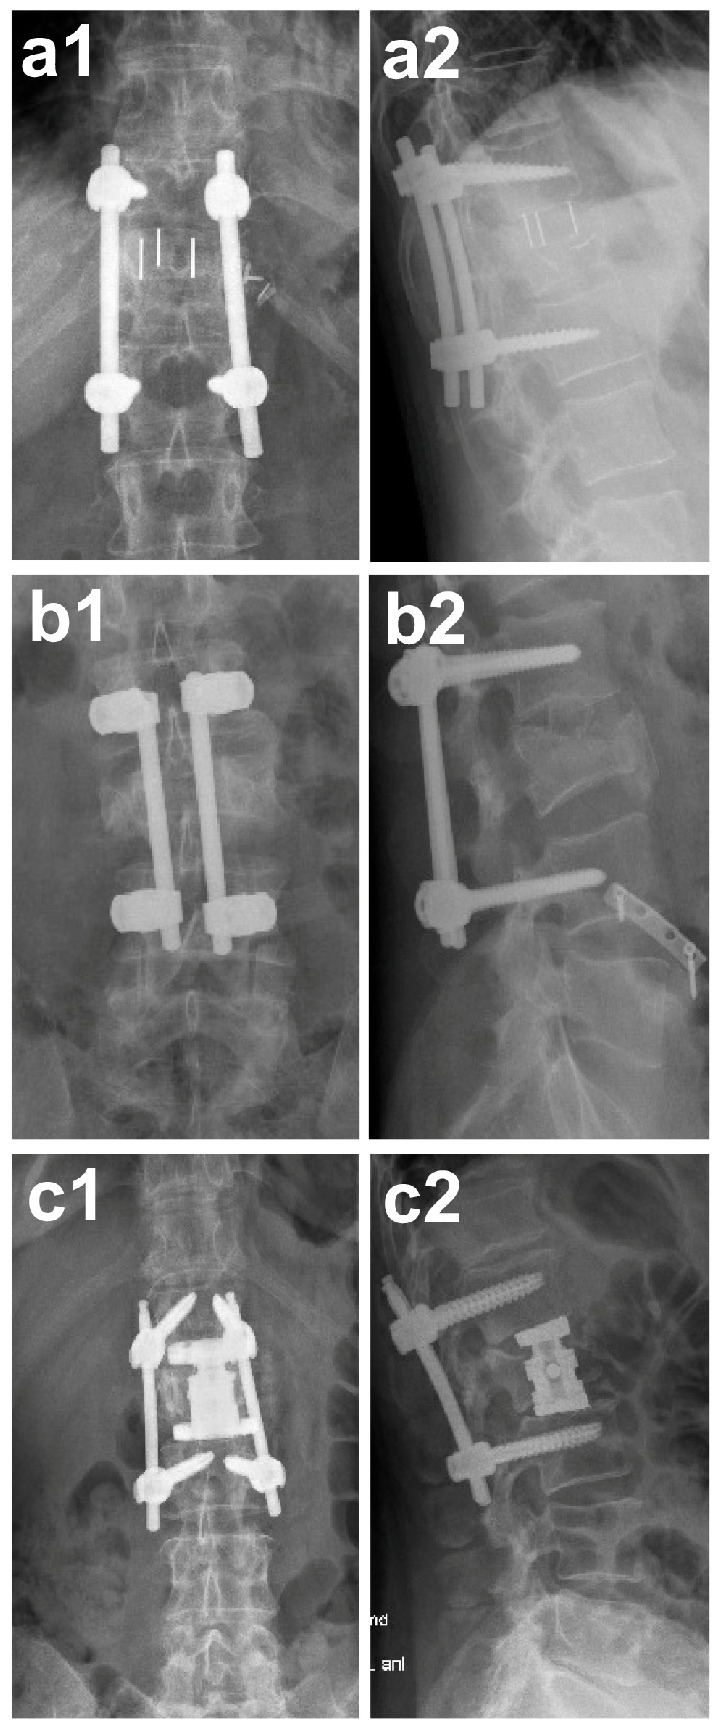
X-ray images of the spine. (a): Postoperative imaging of the spine after cage implantation in anterior–posterior (a1) and lateral projection (a2). (b): Postoperative imaging of the spine after autologous pelvic bone graft implantation in anterior–posterior (b1) and lateral projection (b2). (c): Postoperative imaging of the spine after vertebral body replacement in anterior–posterior (c1) and lateral projection (c2). Adapted from Jäckle *et al.*, Medicina, 2021, 57, 786 [13]. Distributed under the terms of the Creative Commons Attribution License (CC BY 4.0).
